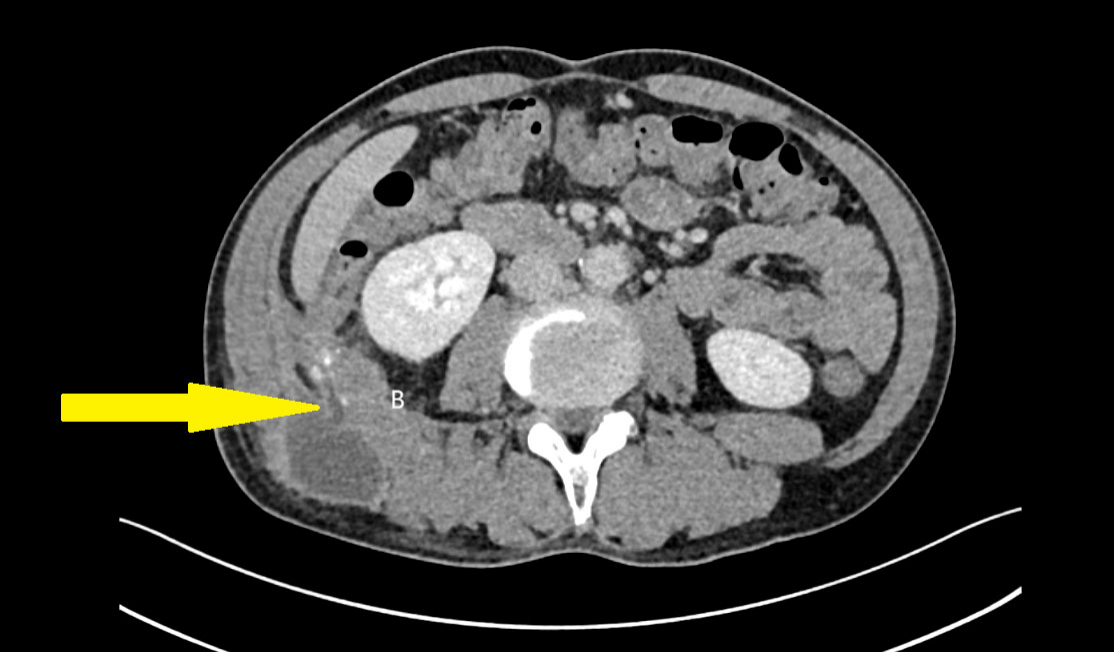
Contrast-enhanced CT showing herniation of the cecum and appendix through the abdominal wall into the lumbar region. The cecum is indicated by the yellow arrow.
